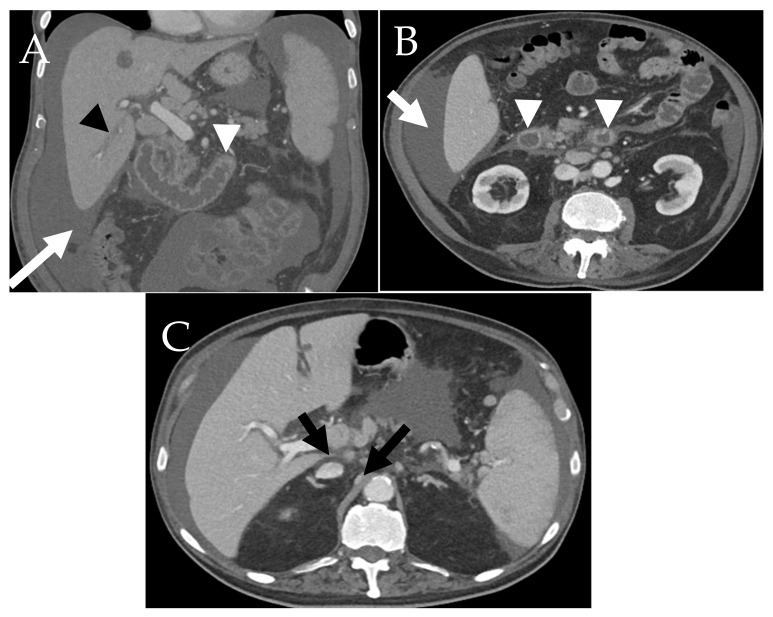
An 80-year-old male with a history of systemic mastocytosis with associated clonal hematological non-mast-cell lineage disease (SM-AHNMD) (A) Coronal contrast-enhanced CT and (B), (C) Axial contrast-enhanced CT scans demonstrate hepatomegaly, periportal edema (black arrowhead), moderate ascites (white arrow), peri-portal and retroperitoneal lymphadenopathy (black arrows), and duodenal and jejunal wall thickening (white arrows heads).
